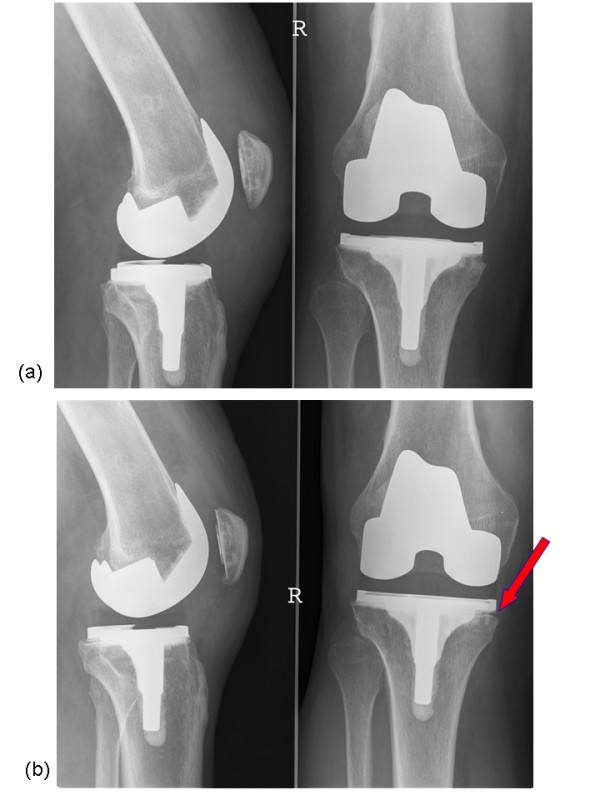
Patient with asymptomatic focal osteolysis of the proximal tibia. (a) Radiographs demonstrating well fixed components and normal bone-implant interface 3 months after surgery. (b) asymptomatic focal osteolysis of the proximal tibia under the prosthetic component at 1 year followup.
